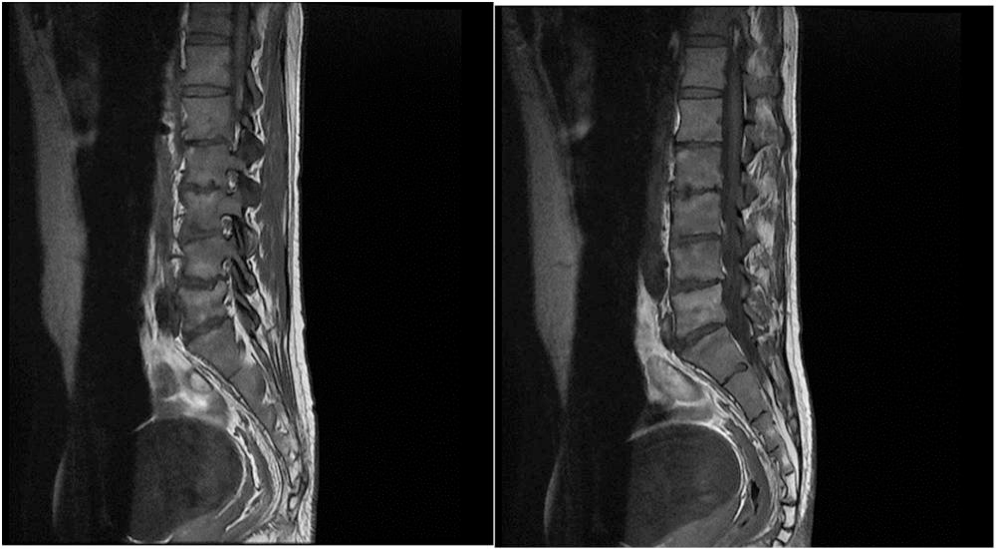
The patient's lumbosacral magnetic resonance imaging scan.
